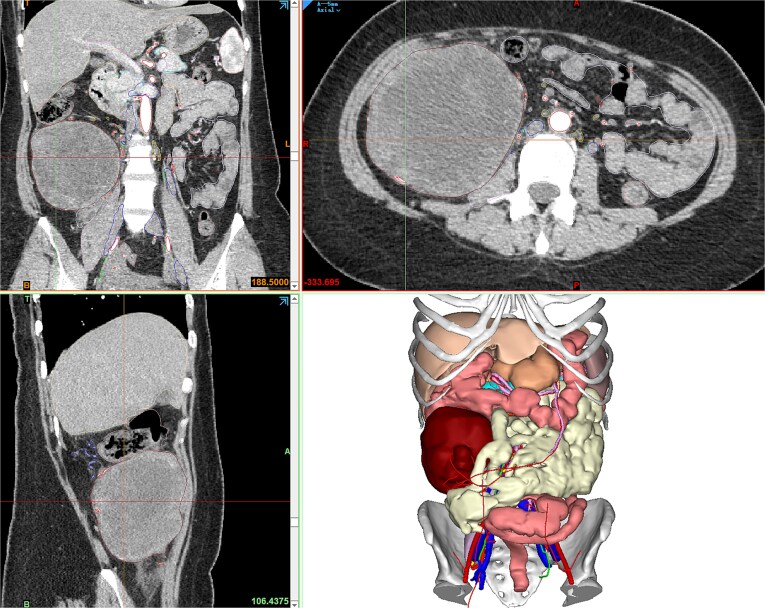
Contrast-enhanced CT showing the retroperitoneal mass, and Mimics23 3D model (tumor: red; ileum; vasculature; 3-Matic®15).
